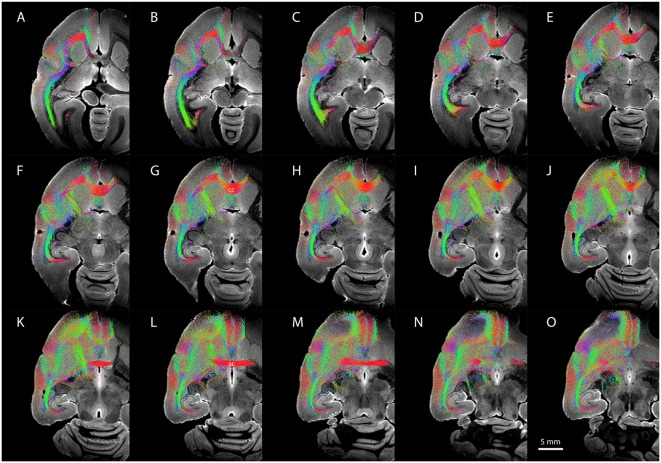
Diffusion-weighted imaging (DWI) tractography showing streamlines from the entire claustrum complex in case CJ197. (A–O) Horizontal sections showing fiber orientation distribution (FOD) for left cerebral hemisphere. Note high streamline density in orbital prefrontal cortex (A–F), insular cortex (D–H), and auditory cortex (F–K) lateral to the corpus callosum (labeled cc in G), and in medial prefrontal cortex (K–O) rostral to the anterior commissure (labeled ac in L).
